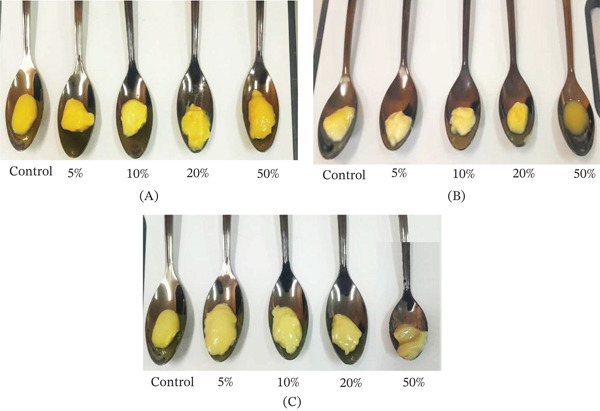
Mayonnaise formulations with different substitution levels of egg yolk. (A) Substitution with proteins from enzymatic‐assisted extraction (EAE), (B) substitution with proteins from ultrasound‐assisted extraction (UAE), and (C) substitution with proteins from ultrasound‐assisted enzymatic extraction (UAEE).
